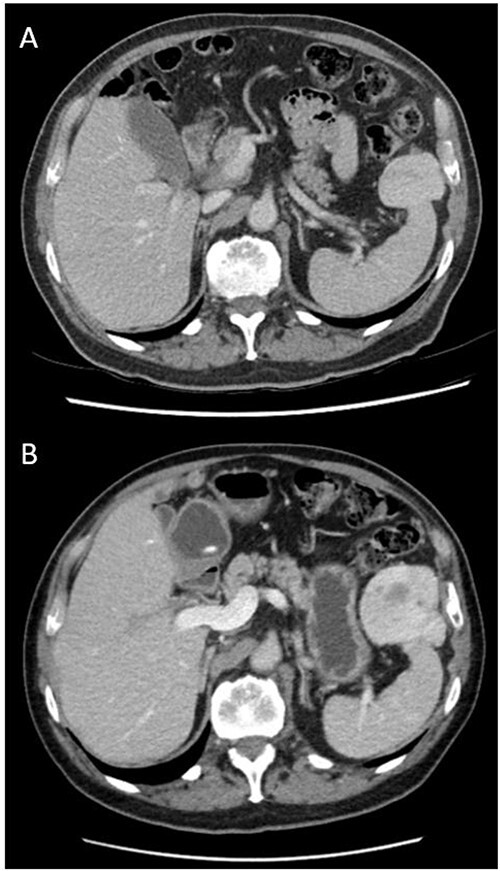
Axial views and comparison of original CT abdomen pelvis to 18-months later. (A) Contrast-enhanced CT abdomen pelvis (arterial phase): axial slice showing solid mass laterally in the left upper quadrant adjacent to the spleen measuring 49 × 39 mm^2^. The spleen is otherwise normal (B) Contrast-enhanced CT abdomen pelvis 18-months after original (arterial phase): Axial slice showing larger mass measuring 64 × 54 × 86 mm^3^ with persistent heterogeneous enhancement and prominent central area of decreased attenuation and peripheral cystic changes.
